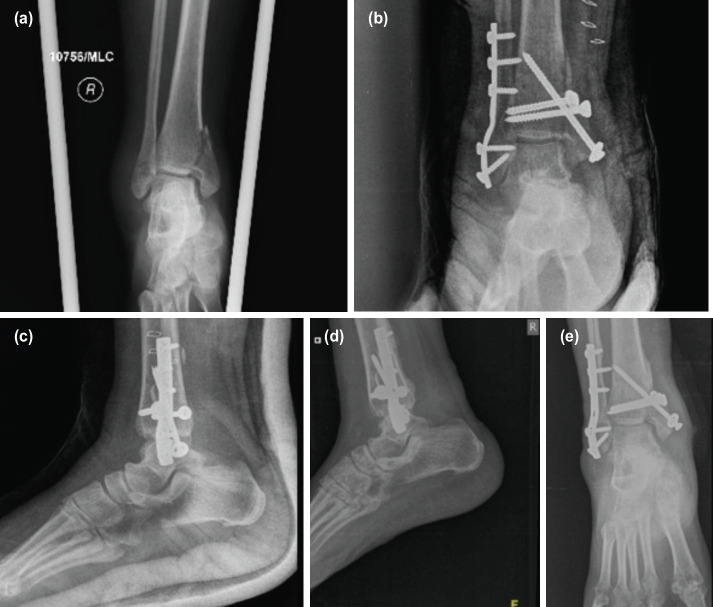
(a) Pre-operative radiograph of a 41-year-old female with bimalleolar ankle fracture who was operated. (b, c) Shows the immediate post-operative radiograph and (d, e) shows medial malleolus non-union at the end of one year follow-up.
